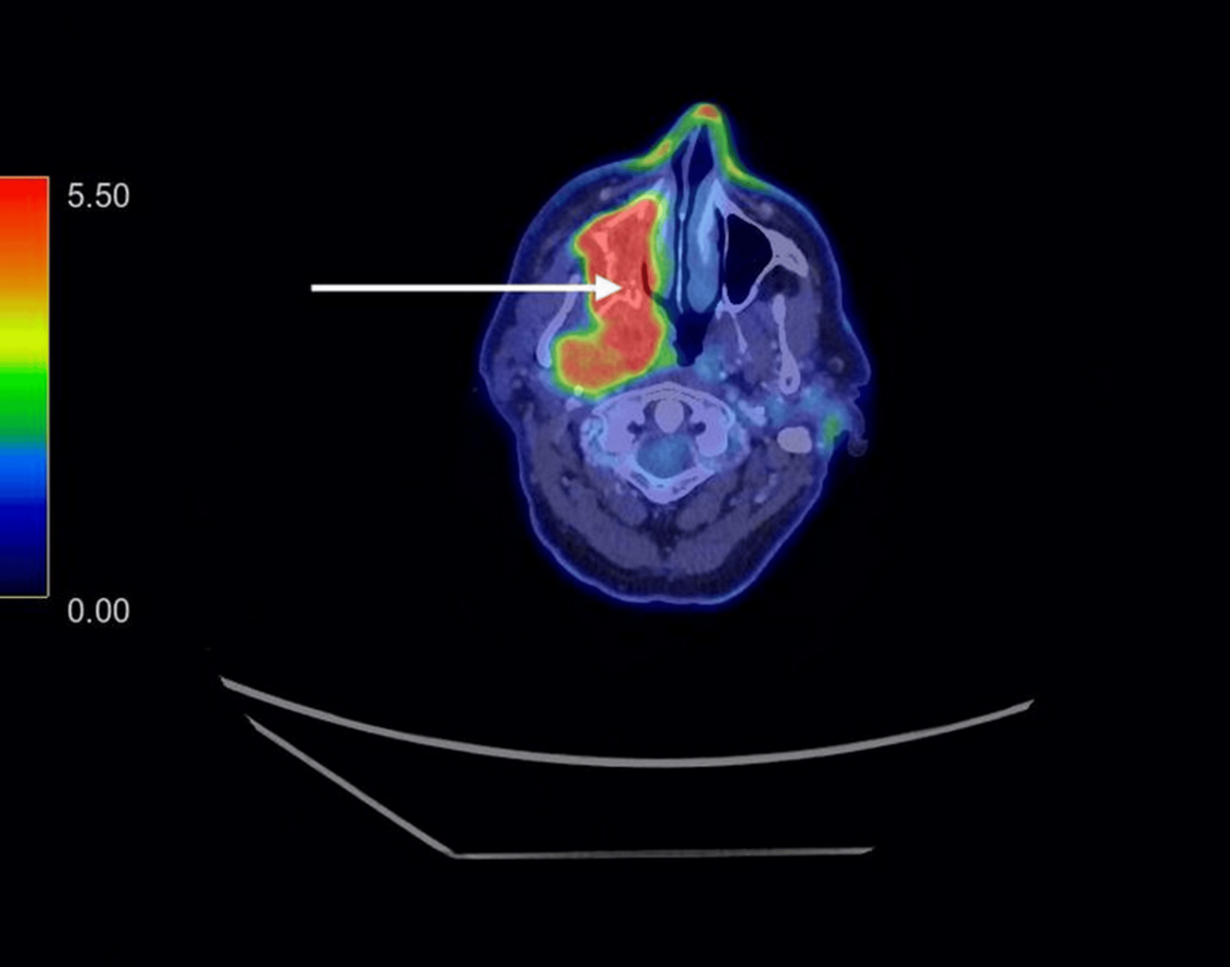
PET-CT showing an extensive right-sided maxillary mass involving the nasopharynx and maxillary sinus, with osseous destruction, pterygoid involvement, and extension into the parapharyngeal space (arrow). PET-CT: positron emission tomography-computed tomography
